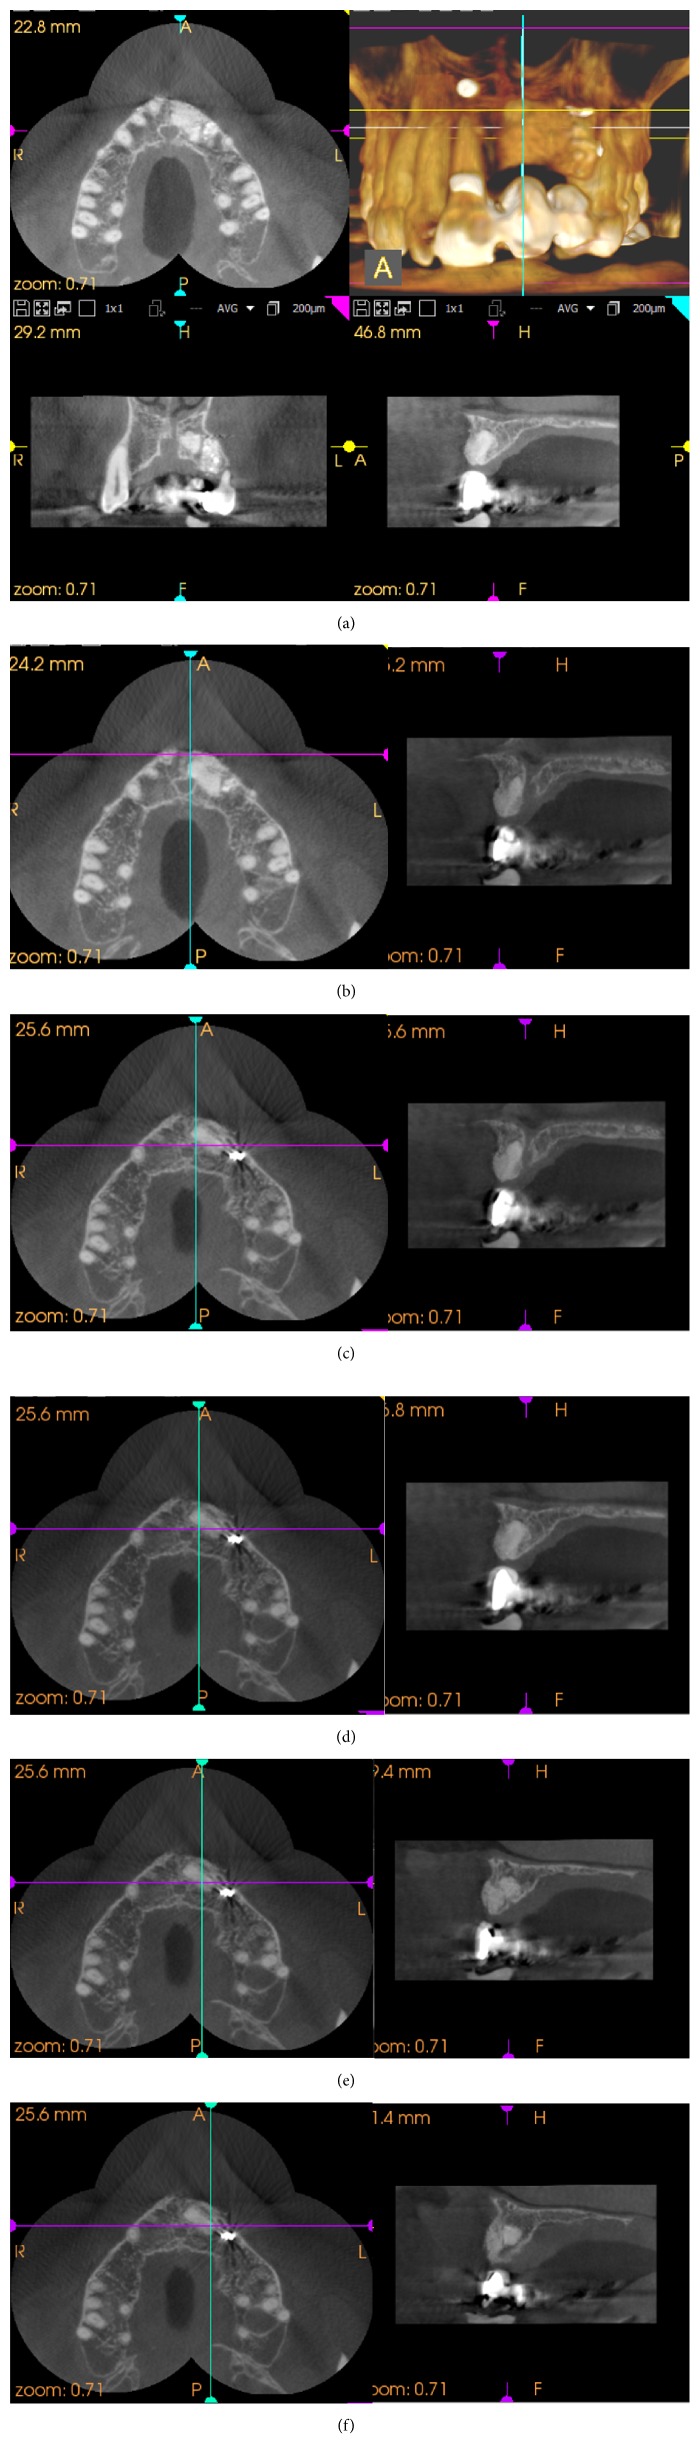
(a) CBCT (Carestream CS9000) imagery taken at 8 months postoperatively displaying the extent of the newly integrating xenograft. (b)–(f) The sagittal views of these films show the well-defined morphology of the aberrant neurovascular canal.
